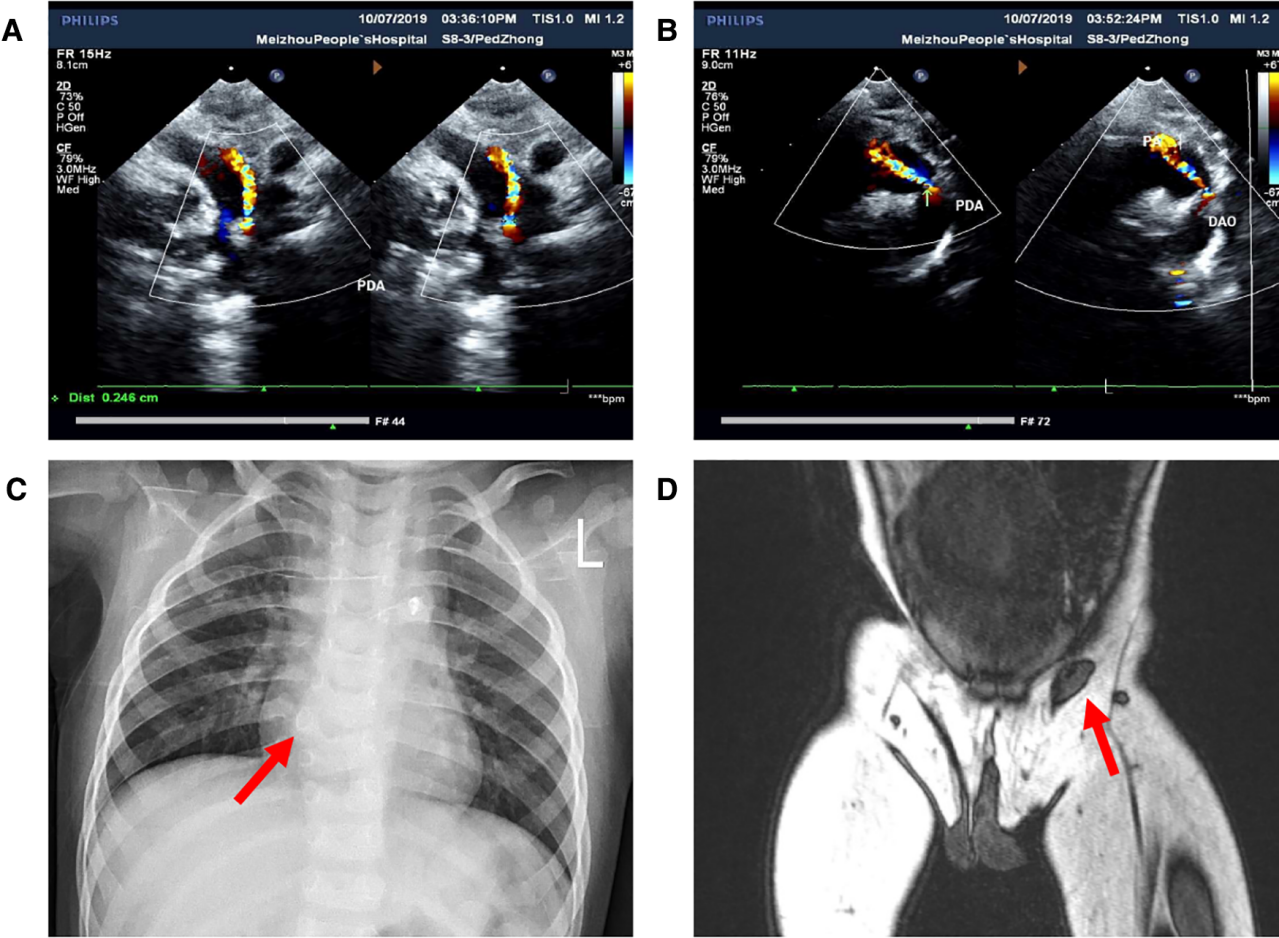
Images of preoperative auxiliary examination. (A,B) Patent ductus arteriosus detected by ultrasonic cardiogram; (C) hemivertebra deformity of the T8 vertebra detected by x-ray; (D) location of the left testis detected by MRI.
